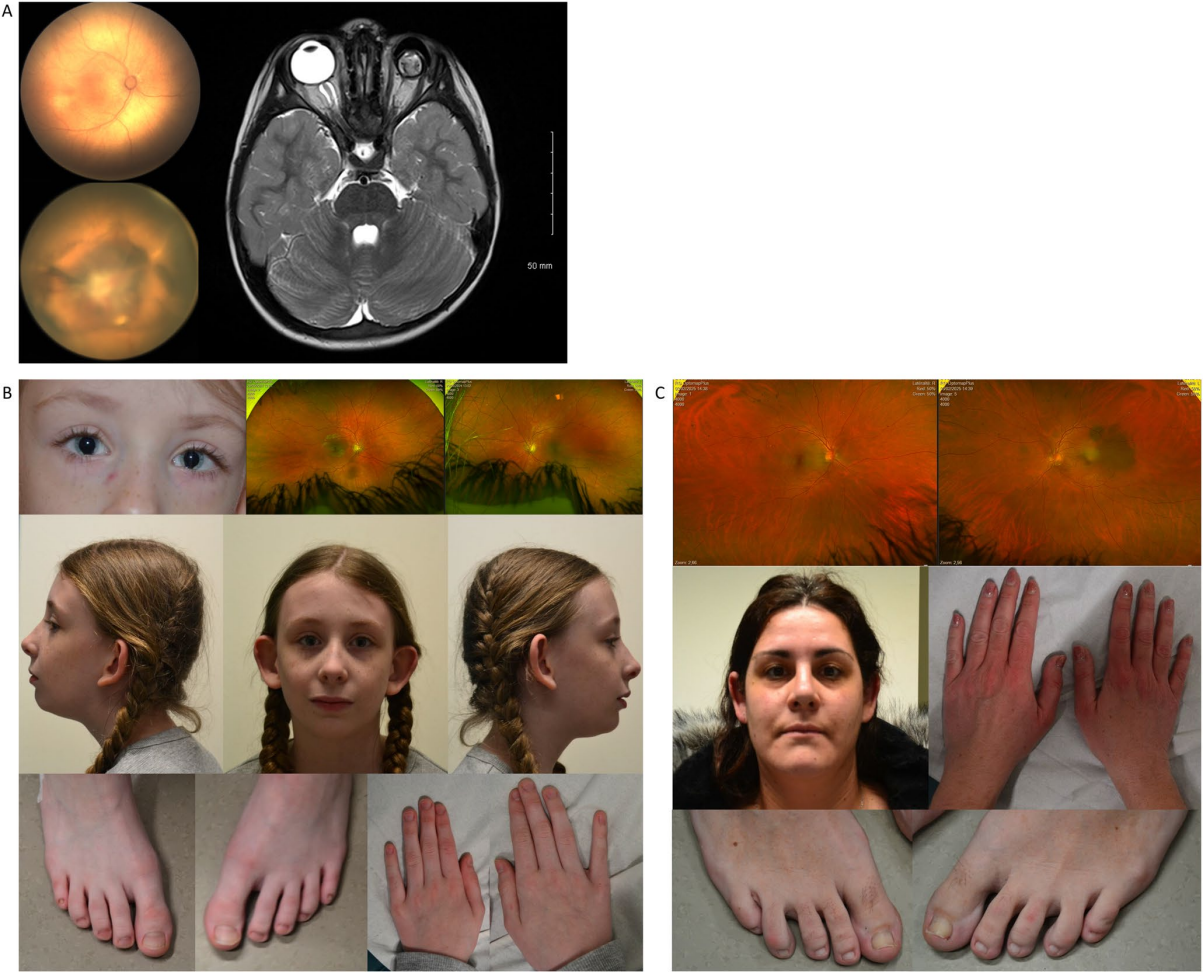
Phenotypic features of individuals carrying a *SMARCA4* LoF variant. (A) Individual 1: Retinophotographs showing a normal right retina (top) and a dystrophic left retina (bottom). Brain MRI demonstrates left‐sided microphthalmia and a hypoplastic left optic nerve. (B) Individual 2A: Dysmorphic and ocular features including iris coloboma with normal retina, prominent ears, long eyelashes, and hypoplastic fifth toes. (C) Individual 2B: Normal clinical and ophthalmological examination.
